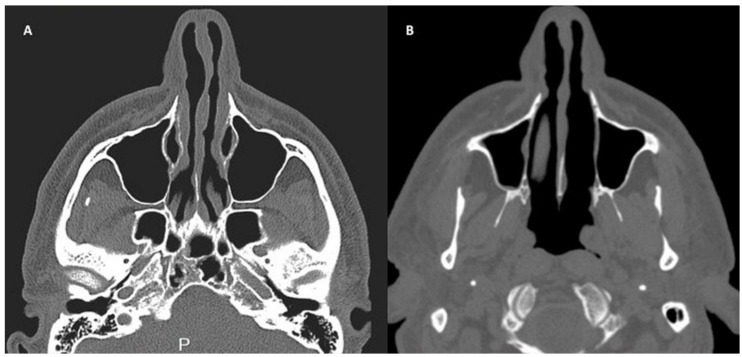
(A) CT scans show the pre-operative situation with bilateral soft tissue defect of nasal tip and cartilage collapse. In addition, nasal septum deviation was detected. (B) CT scans of the same area after 12 months show the regenerated site in the post-operative image with soft tissue volume improvement and the correction of the nasal septum deviation.
